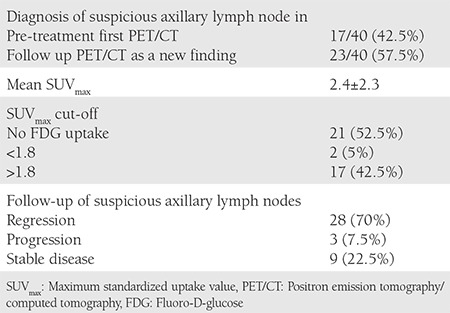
Positron emission tomography/computed tomography features, maximum standardized uptake value values, and results of a suspicious axillary lymph node imaging
